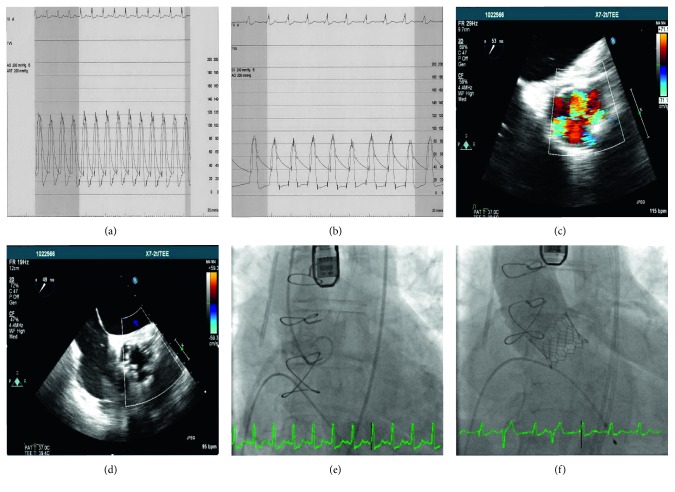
(a) Pre-TAVI simultaneous aortic and left ventricular pressures demonstrating equalization of Ao diastolic LV end-diastolic pressures. (b) Post-TAVI hemodynamics revealing normal diastolic LV-Ao gradient and no systolic LV-Ao gradient. (c) Pre-TEE image of the bioprosthetic aortic valve during diastole shows three large regurgitant jets. (d) Post-TEE short axis of the TAVI valve during diastole shows absence of regurgitant flow. (e) Pre-TAVI aortogram shows 4+ aortic insufficiency. (f) Post-TAVI aortogram shows no aortic insufficiency and acceptable implantation depth. TAVI = transcatheter aortic valve replacement; Ao = aortic; LV = left ventricular; TEE = transesophageal echocardiogram.
